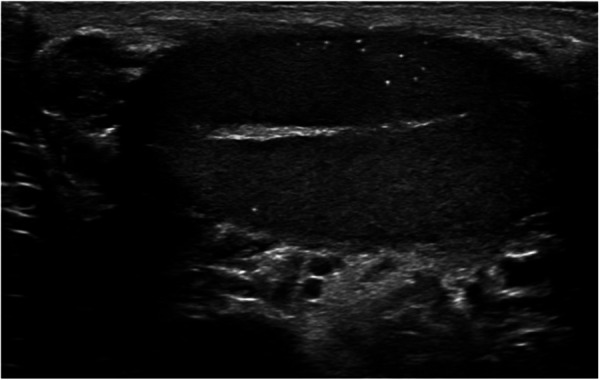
An ultrasound of a patient with TM showing the conditions characteristic punctate calcifications.
